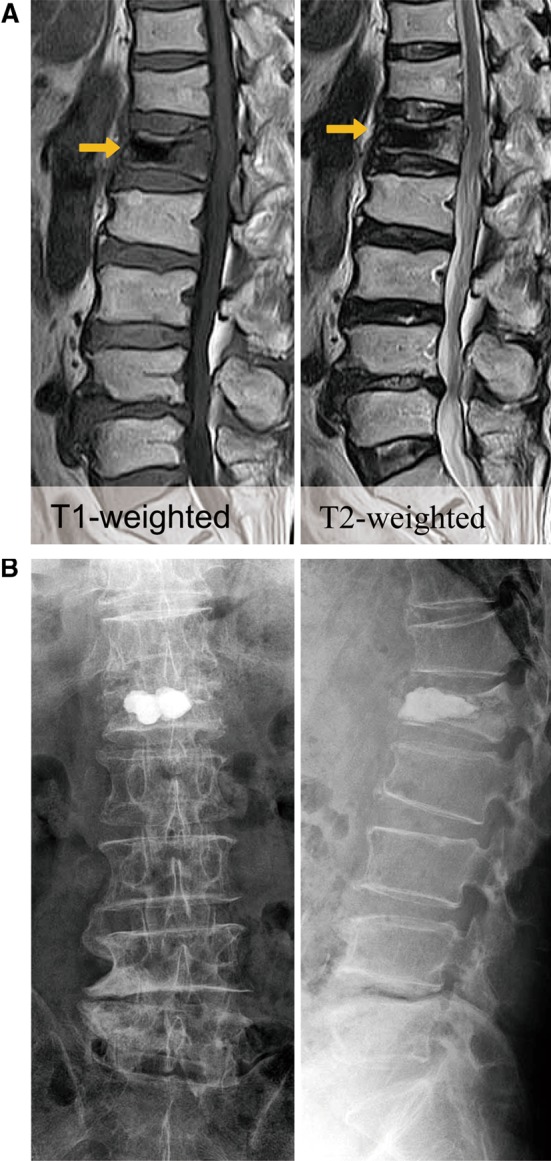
(Case 1) a Sagittal T1- and T2-weighted images showing collapsed vertebral body with formation of a cavitary lesion at the L1 level. b Radiograph of the spine after BKP reveals effective filling of cystic fracture cavity
